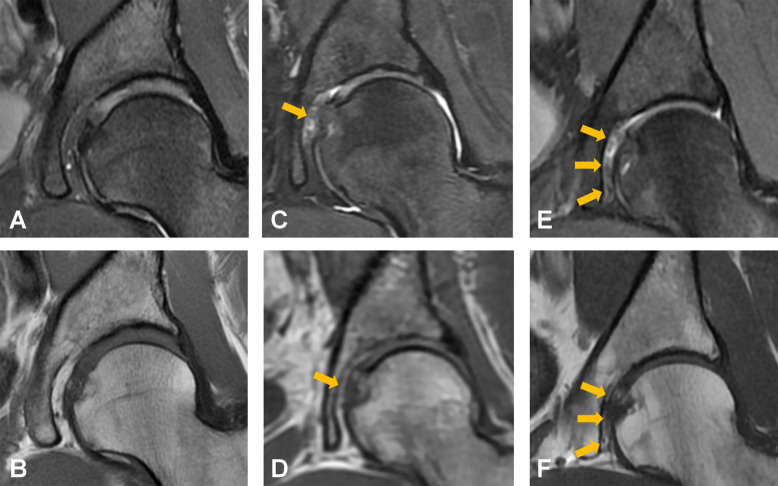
Grading of pulvinar signal abnormalities on coronal T1-weighted and intermediate-weighted fat-suppressed images. Grade 0 (A, B): normal appearance of the pulvinar. Grade 1 (C, D): centrally located pulvinar signal abnormalities with < 50% fat replacement (arrows). Grade 2 (E, F): extensive pulvinar signal abnormalities with > 50% fat replacement (arrows)
